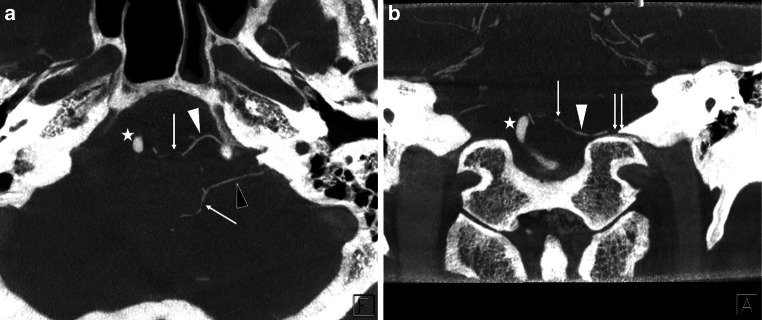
A representative case of the jugular foramen (JF) bridging vein (BV). Axial (a) and coronal (b) view of the slab MIP images from contrast-enhanced cone-beam CT show that the transverse medullary vein (*arrow* in (a) and (b)) drains into the inferior petrosal sinus (*double*
